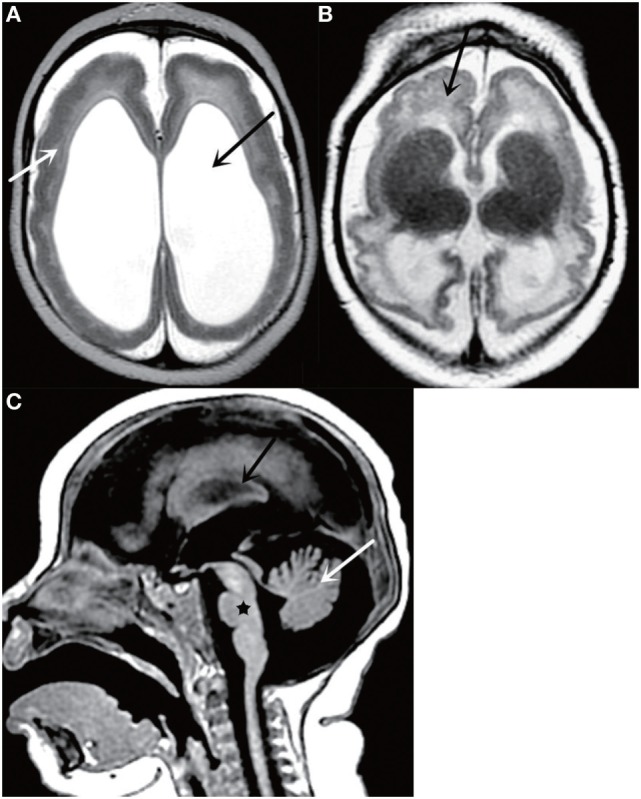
Axial T2-weighted MR image (A) shows a thickened cortex (white arrow) and ventriculomegaly (black arrow); Axial T2-weighted MR image (B) shows cortical malformations (black arrow); Sagittal T2-weighted MR image (C) shows cerebellar atrophy (white arrow), corpus callosum abnormalities (black arrow), and brainstem dysplasia (black star). All figures are courtesy of Lara Brandão M.D., Rio De Janeiro, Brazil.
